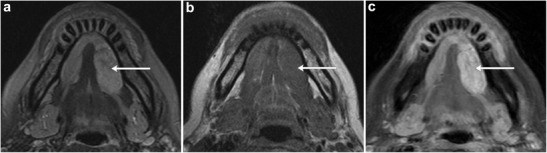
Adenoid cystic carcinoma of the sublingual gland. a Axial T2-weighted and b T1-weighted MR images of the sublingual space demonstrate a lobulated high T2 signal and low T1 signal lesion enlarging and distorting the left sublingual gland (*arrow*). c There is diffuse enhancement on the axial post-contrast T1-weighted MR image
